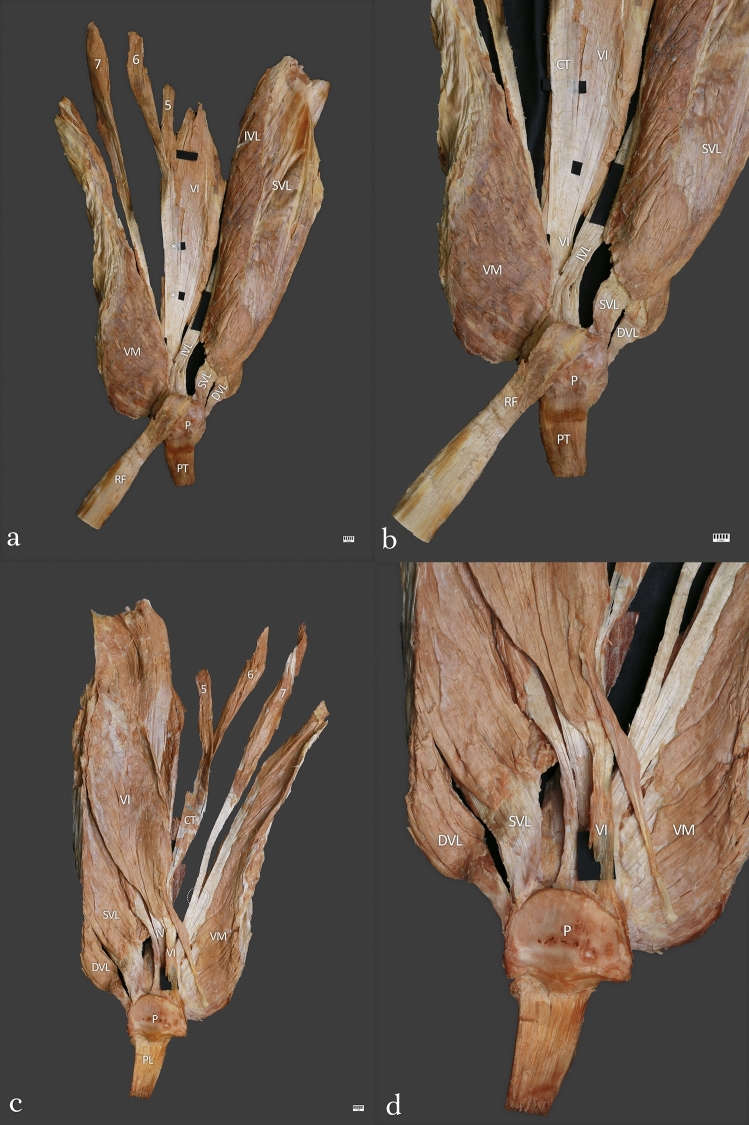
a Type IV of the quadriceps femoris tendon. Anterior view. Left side. *RF* rectus femoris, *P* patella, *PT* patella tendon, *VM* vastus medialis, *SVL* superficial part of the vastus lateralis, *IVL* intermediate part of the vastus lateralis, *DVL* deep part of the vastus lateralis, *VI* vastus intermedius, *5* fifth head of the quadriceps femoris, *6* sixth head of the quadriceps femoris, *7* seventh head of the quadriceps femoris *common tendon for the fifth and sixth head of the quadriceps femoris. b Type IV of the quadriceps femoris tendon. Enlarged photo. Anterior view. Left side. *P* patella, *PT* patella tendon, *RF* – rectus femoris, *VI* vastus intermedius, *CT* common tendon, *IVL* intermediate part of the vastus lateralis, *SVL* superficial part of the vastus lateralis, *DVL* deep part of the vastus lateralis. c Type IV of the quadriceps femoris tendon. Posterior view. Left side. *P* patella, *PT* patella tendon, *VI* -vastus intermedius, *SVL* superficial part of the vastus lateralis, *IVL* intermediate part of the vastus lateralis, *VM* vastus medialis, *CT* common tendon, *5* fifth head of the quadriceps femoris, *6* sixth head of the quadriceps femoris, *7* seventh head of the quadriceps femoris. d Type IV of the quadriceps femoris tendon. Enlarged photo. Posterior view. Left side. *DVL* deep part of the vastus lateralis, *SVL* superficial part of the vastus lateralis, *VI* vastus intermedius. *VM* vastus medialis
